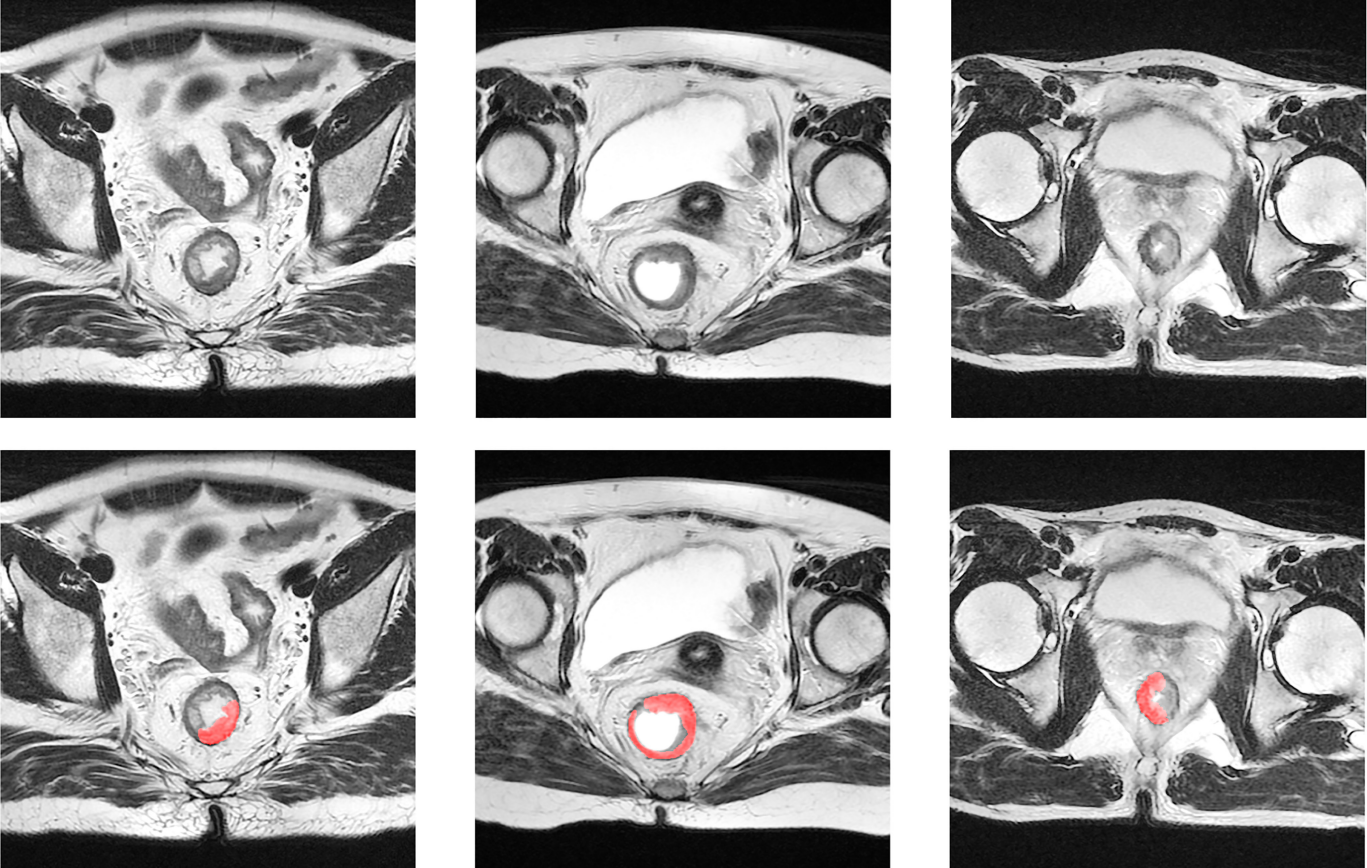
Examples of suspicious region. The first row is the original T2-w MRI, and the second row displays the delineation of the suspicious region in red color.
